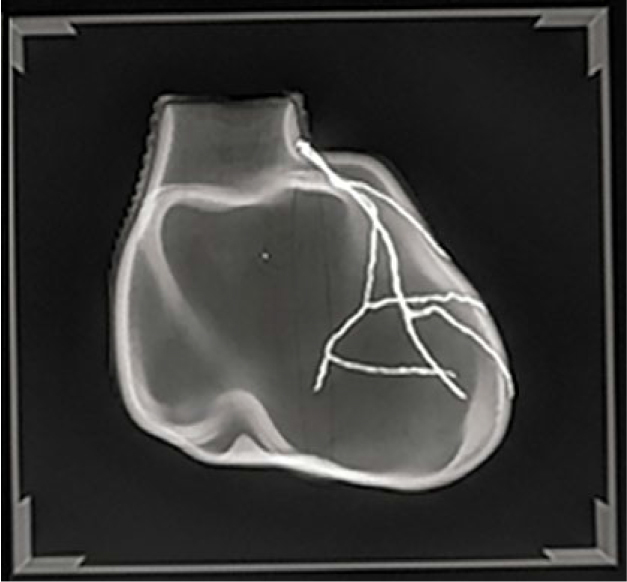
Radiograph of the heart model with vessels drawn by a mixture of UV gel and metallic powder.
